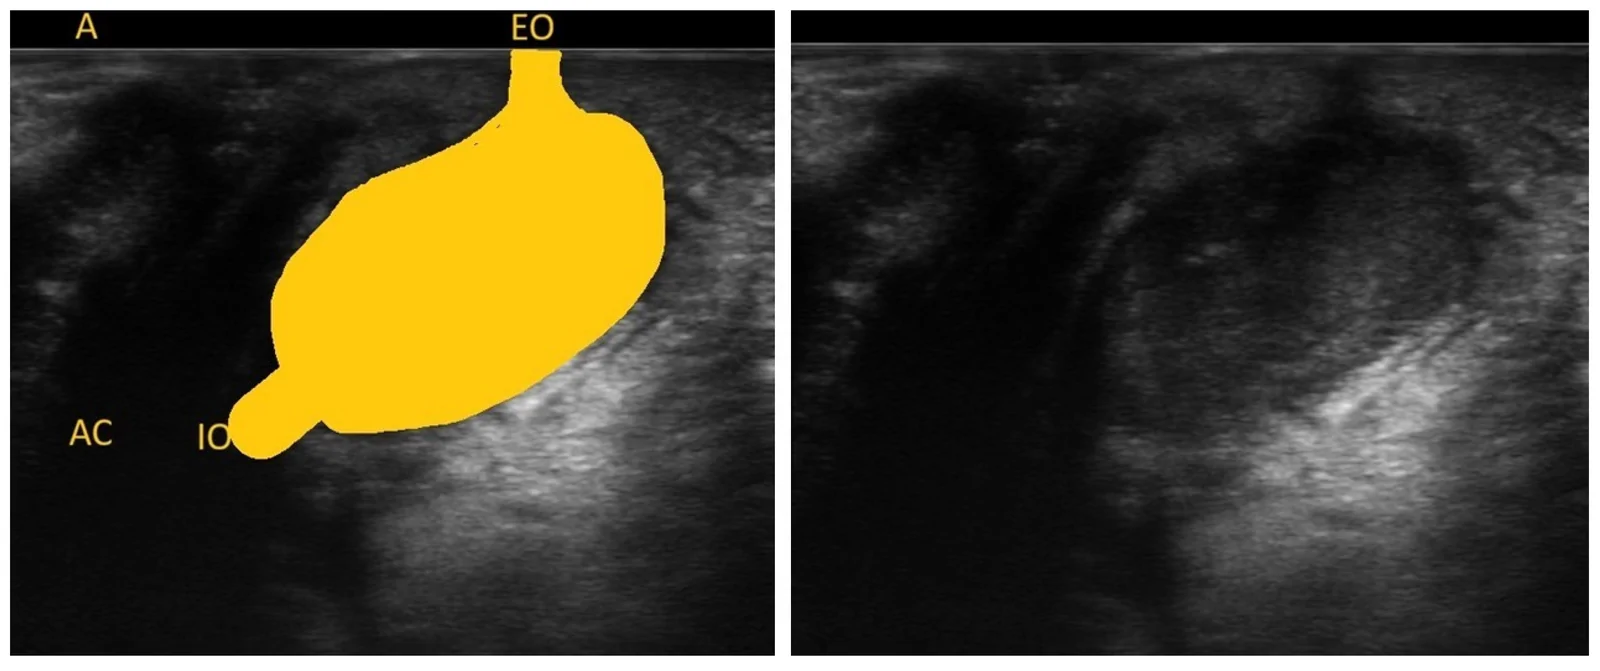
An ultrasound image obtained using a superficial linear probe (7.5 MHz) shows a right residual perianal abscess of 24 × 14 mm in maximal dimensions, with an obvious external opening (EO) and two internal openings (IO) into the anal canal (AC) located 19 mm and 16 mm from the anal margin (A). The other IO requires an additional scan to be observed. The left image shows the abscess (thick yellow line), EO, and IO. The right image is the corresponding unannotated scan.
